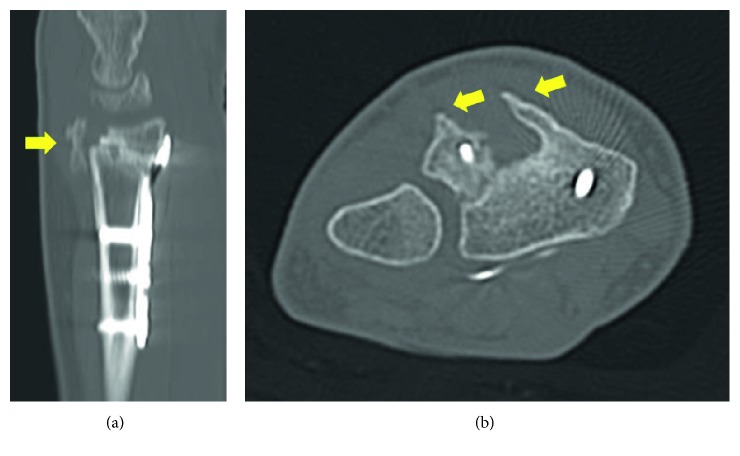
CT scan performed at 6-month follow-up. The arrows show dorsal bone spur.
